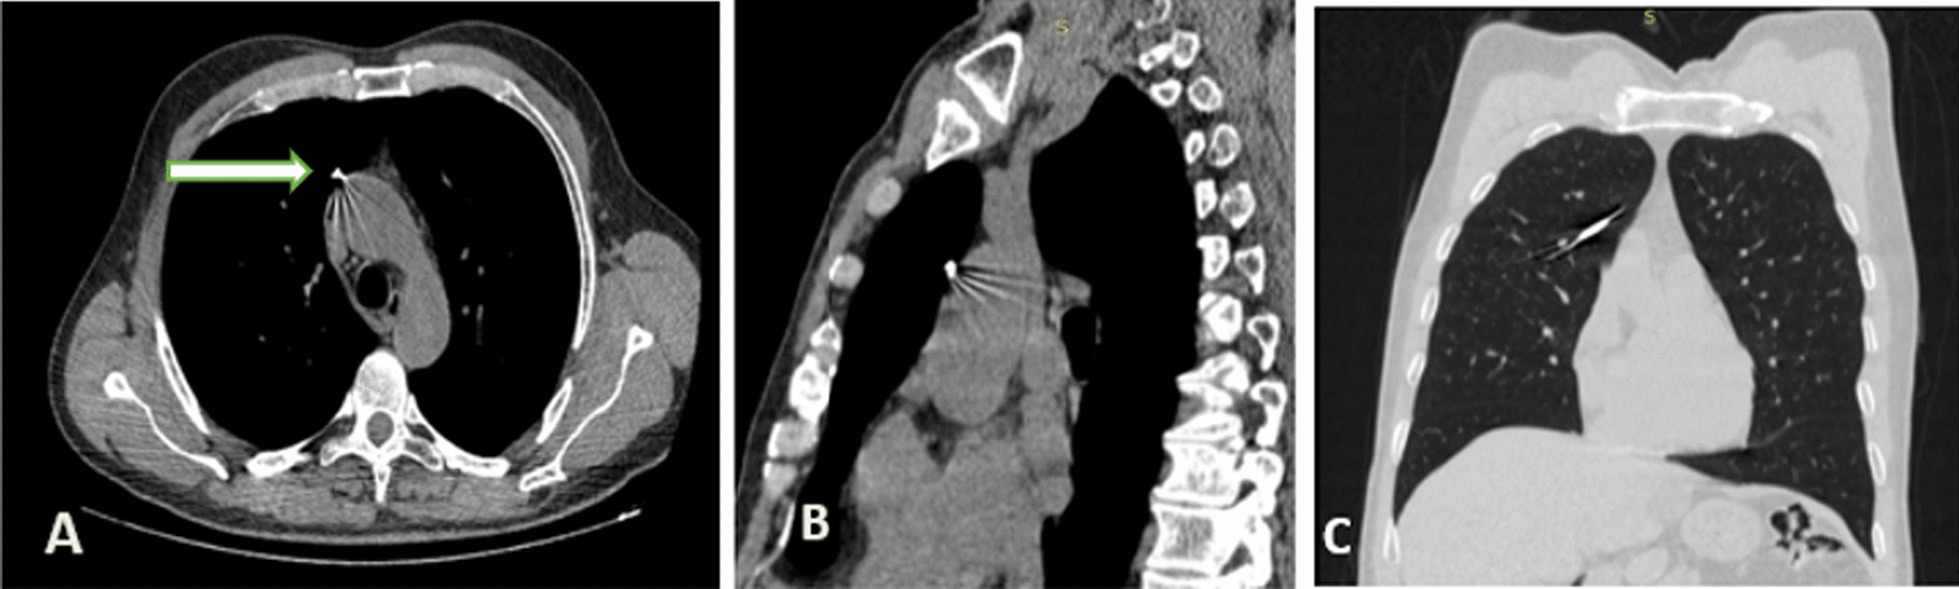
A–C Chest Computed tomography showing the wire in the upper right lobe adjacent to ascending aorta (white arrow shows well the pin)
